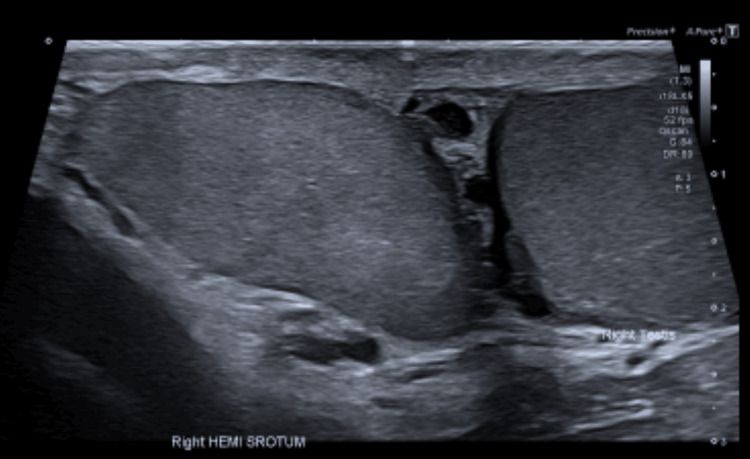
Scrotal ultrasound demonstrating two right-sided testes
